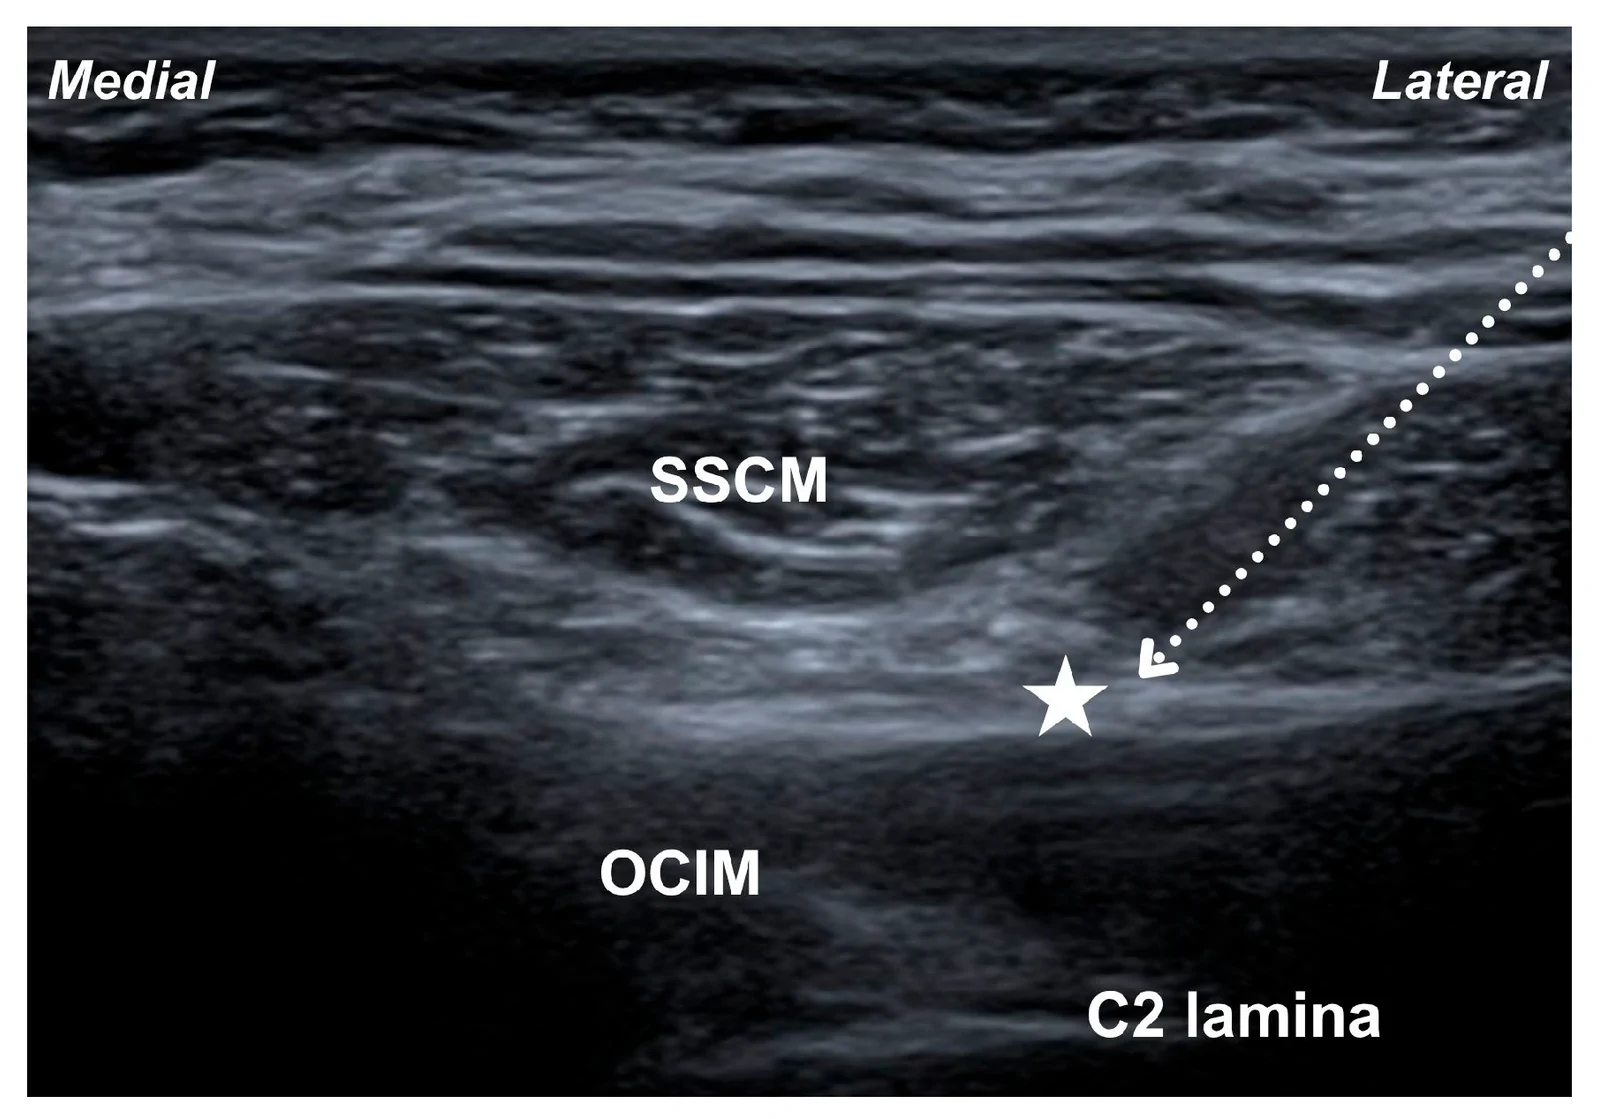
Ultrasound-guided proximal greater occipital nerve pulsed radiofrequency (GON-PRF) at the C2 level. The ultrasound image demonstrates the in-plane lateral-to-medial approach targeting the greater occipital nerve (white asterisk), located between the semispinalis capitis muscle (SSCM) and the obliquus capitis inferior muscle (OCIM), adjacent to the C2 lamina. The dotted line indicates the trajectory of the radiofrequency cannula. SSCM, semispinalis capitis muscle; OCIM, obliquus capitis inferior muscle.
